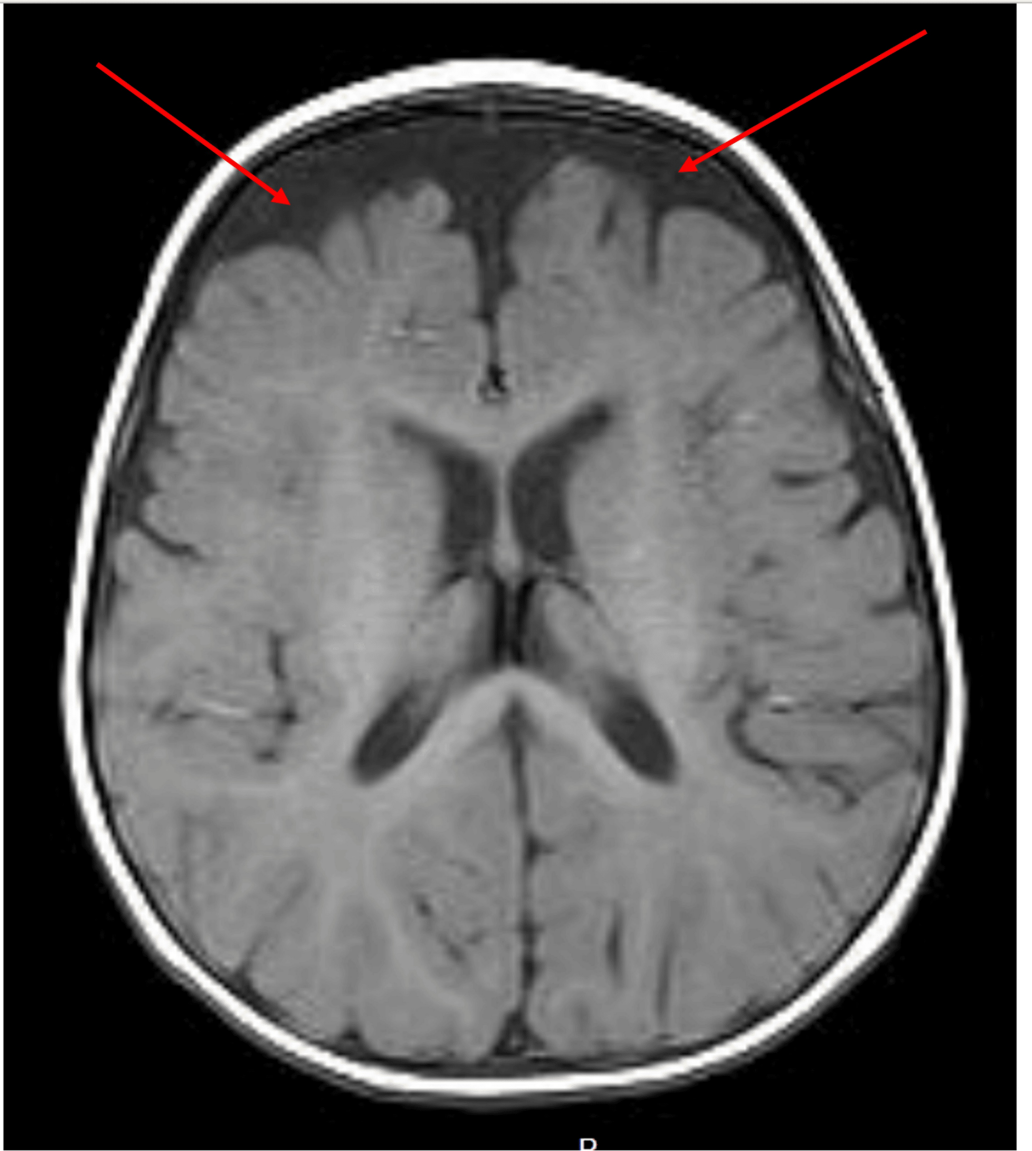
T1 sequence brain MRI - axial view The image shows increased EA-CSF volume in the subarachnoid spaces over the bilateral frontal lobes EA-CSF: extra-axial cerebrospinal fluid; MRI: magnetic resonance imaging
